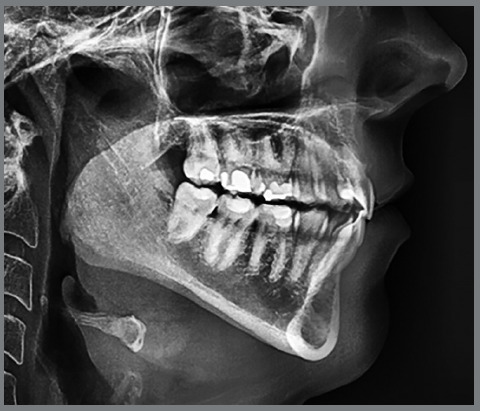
Lateral cephalometric radiograph showing a patient presenting a posterior open bite (lack of posterior occlusal contact).
